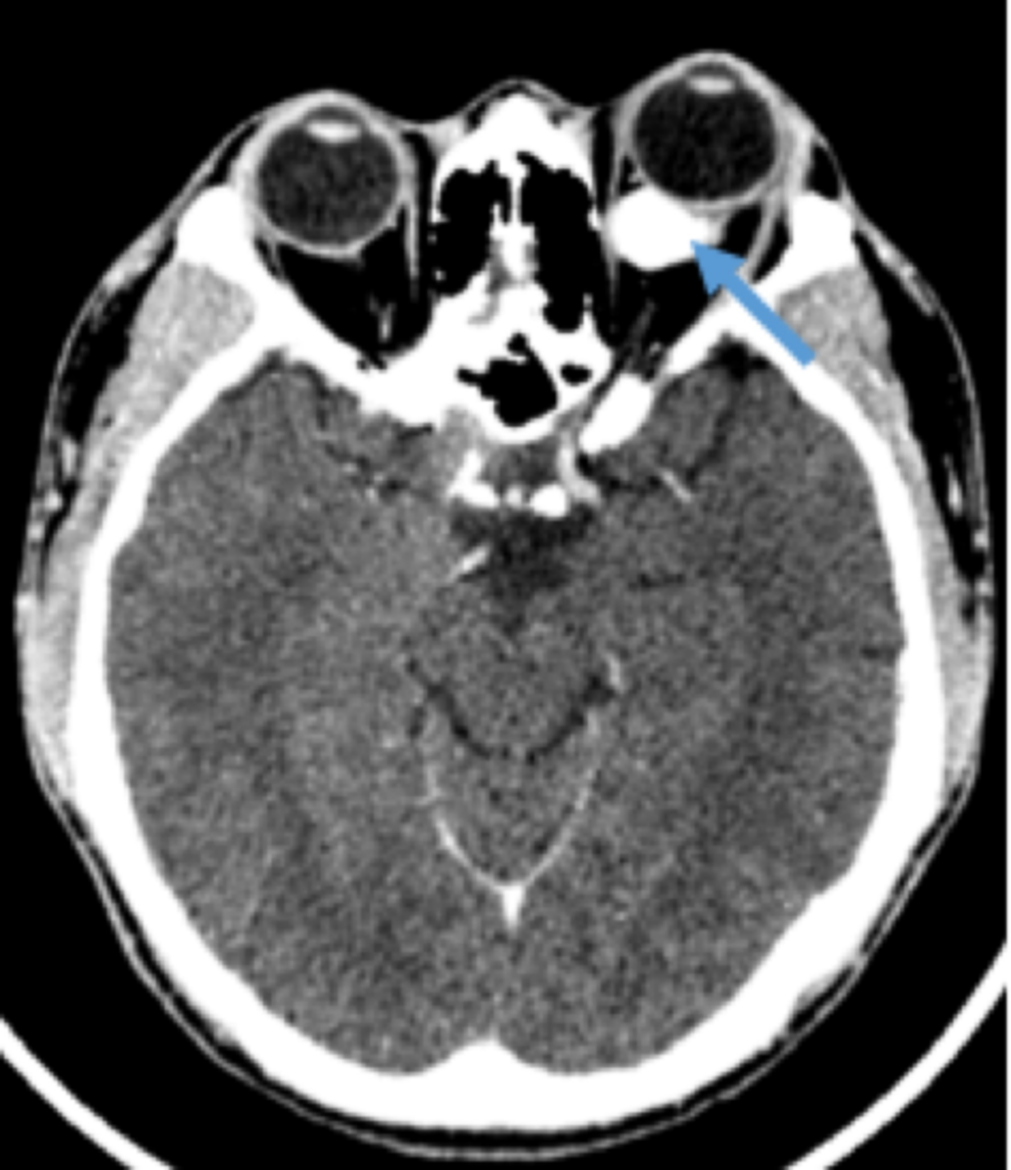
Axial view of a computed tomography image showing a well defined enhancing intraconal mass
